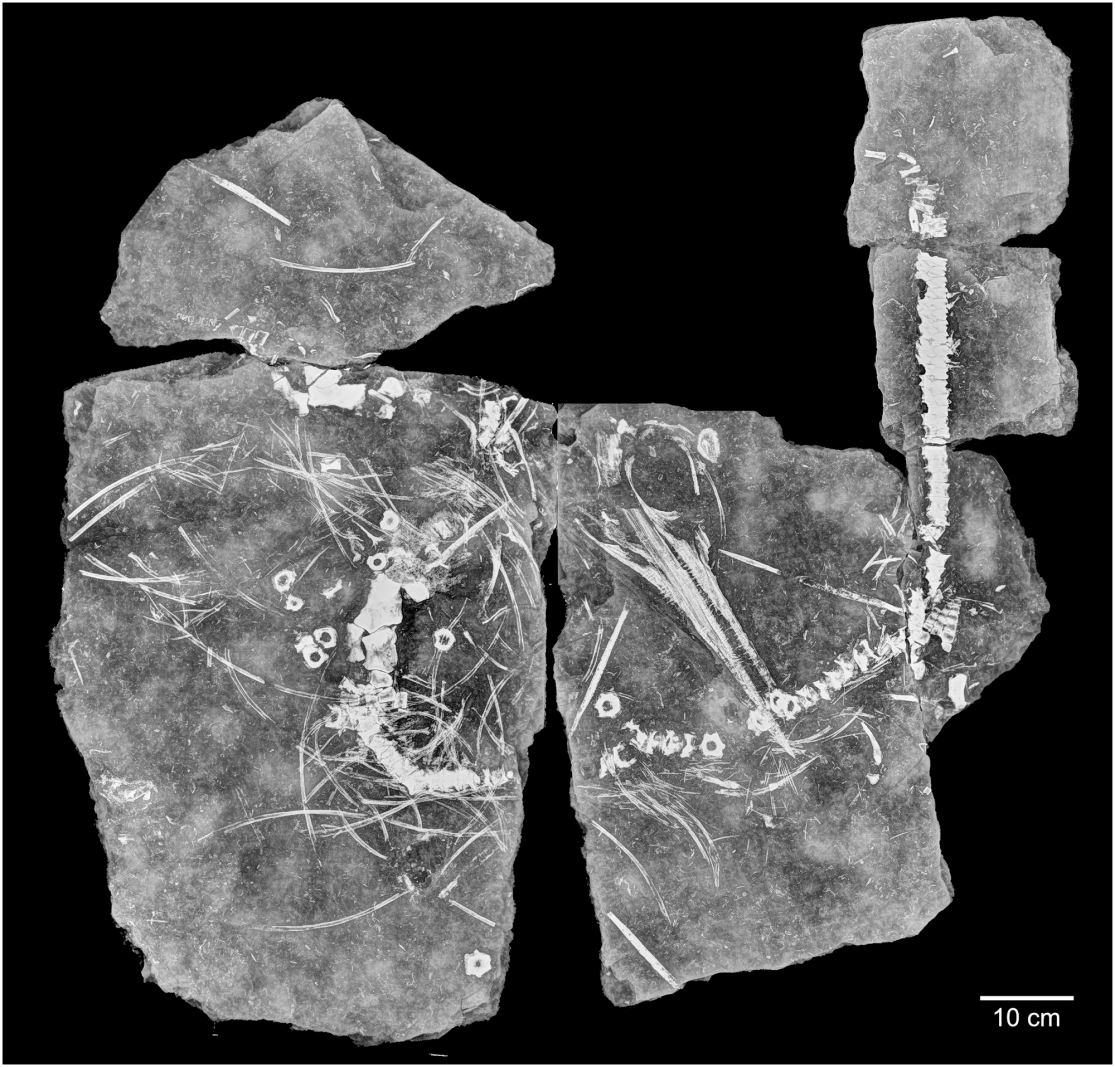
Radiograph image of PMO 219.250.
